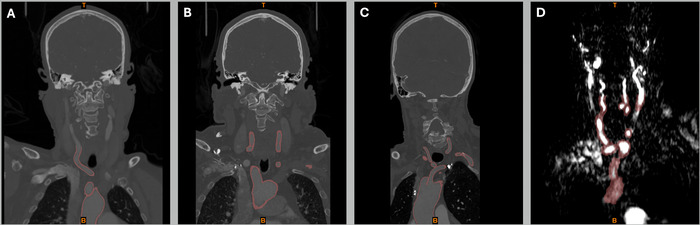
Coronal DICOM (Digital Imaging and Communications in Medicine) images of the aortic arch vasculature from 4 patients: (A) case 1, (B) case 2, (C) case 3, and (D) case 4. Each patient had an abnormal aortic arch with tortuous arteries that complicated neurointerventional procedures. Cases 1, 2, and 3 underwent computed tomography angiography; case 4 underwent magnetic resonance angiography because the patient was allergic to iodine contrast media.
